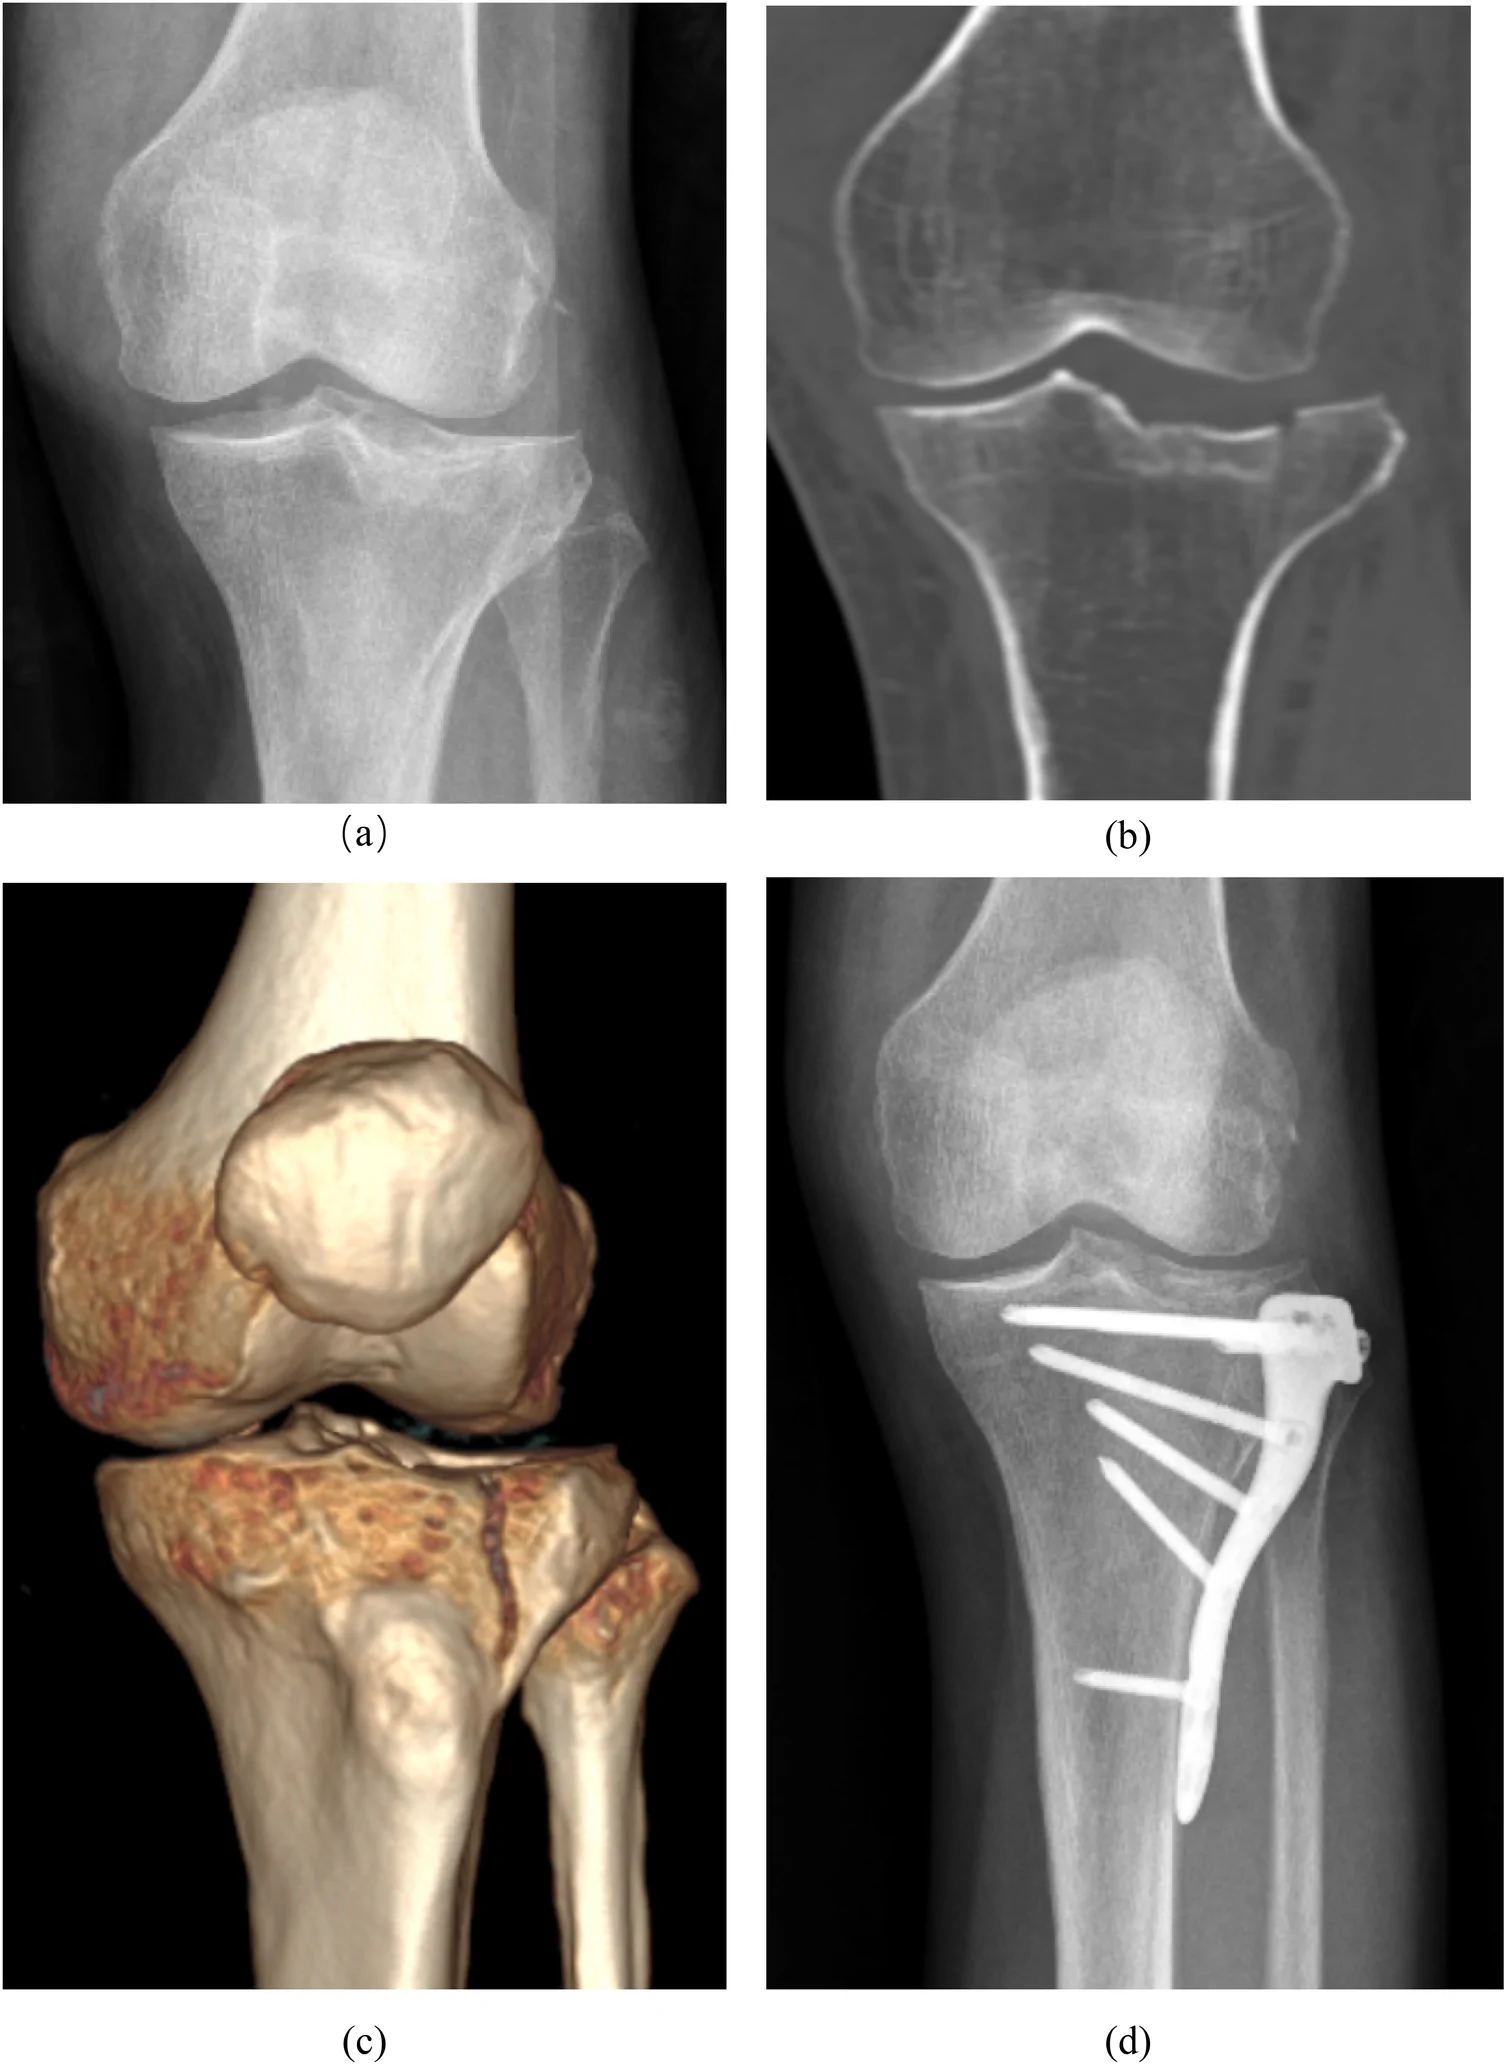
Pre-operative and post-operative imaging of Schatzker type-II tibial plateau fracture. (a) Pre-operative anteroposterior radiograph of knee; (b) Coronal CT scan showing depressed tibial plateau fracture; (c) Three-dimensional CT reconstruction demonstrating fracture morphology; (d) Post-operative anteroposterior radiograph after open reduction and internal fixation with lateral rafting plate and screws.
